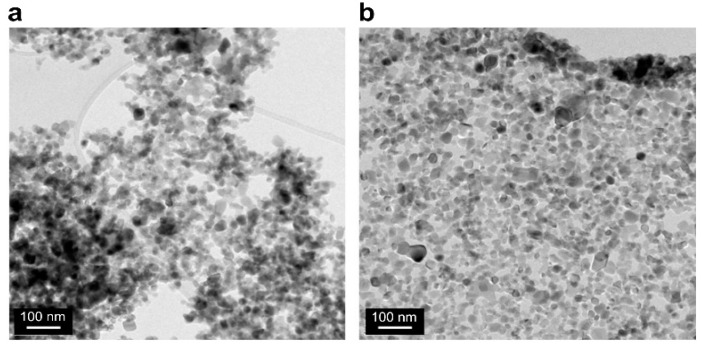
TEM images before (a) and after (b) ultrasonic dispersion.
